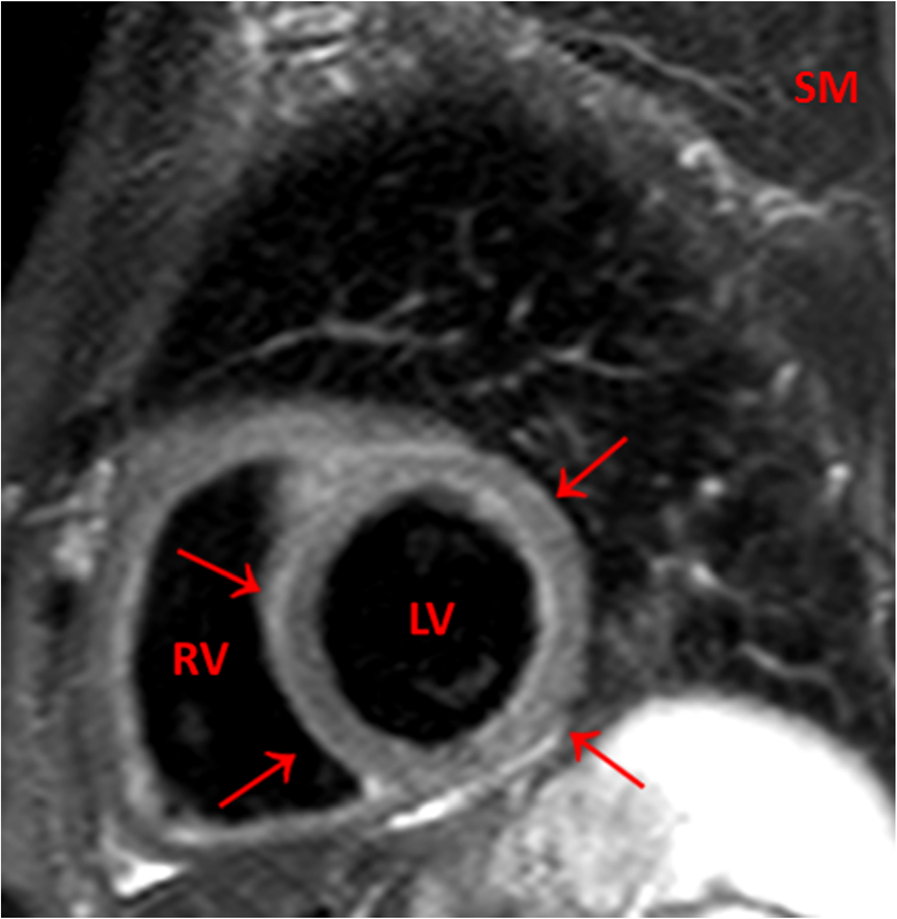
An exemplary T2-weighted (STIR) image of a 57 year old female patient with a viral respiratory viral infection 3 weeks ago and exercise induced PVBs. Globally enhanced signal intensity of the myocardium of the left ventricle (*arrows*) compared to skeletal muscle. The ratio of signal intensity between myocardial and skeletal muscle was elevated (2.26). LV: Left ventricle. RV: Right ventricle. SM: Skeletal muscle
